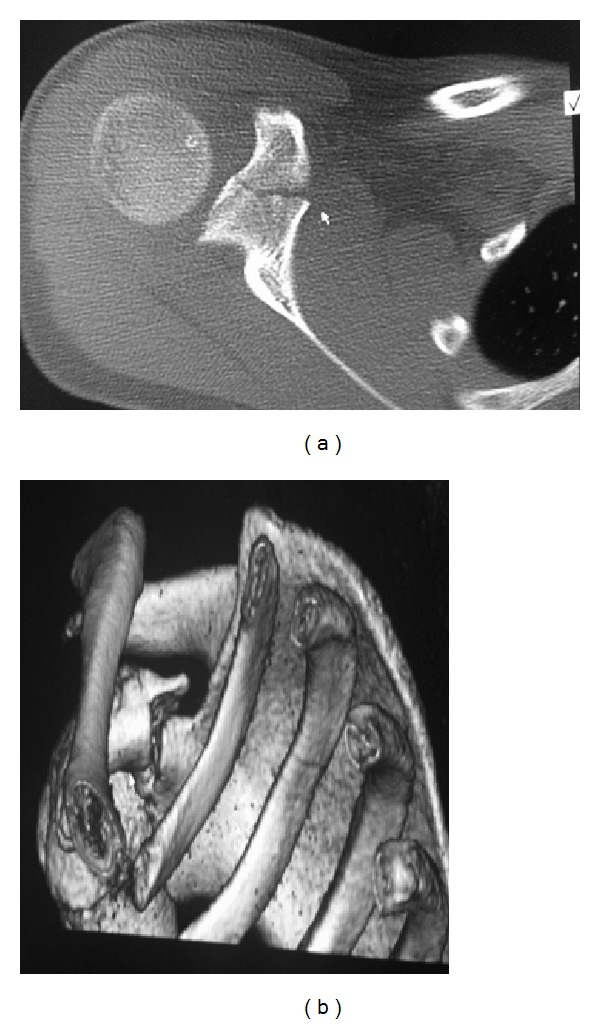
Case B: axial CT scan and CT 3D reconstruction.
